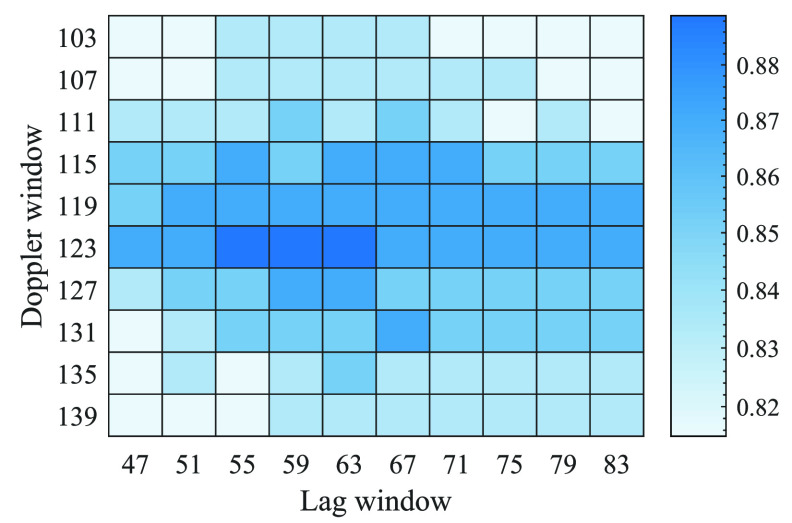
Proposed system performance as function of TFD parameters: Doppler and lag window length. Testing results using a nested 5-fold cross-validation within the 1st leave-one-subject-out iteration of the development dataset.
